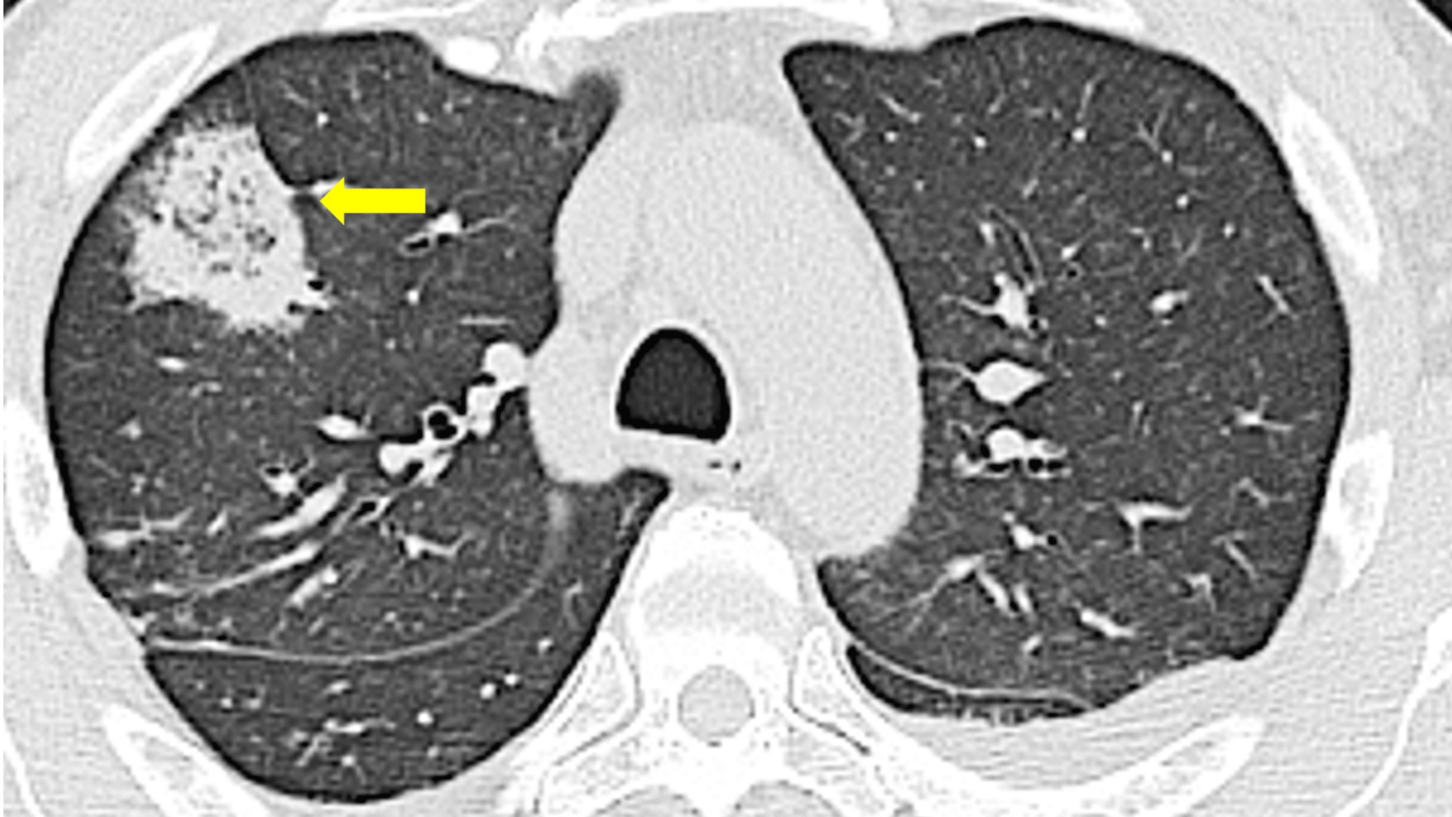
Chest computed tomography (CT) image on hospital day 1 demonstrating a mass with reversed halo sign in the right upper lobe of the lung (yellow arrow).
